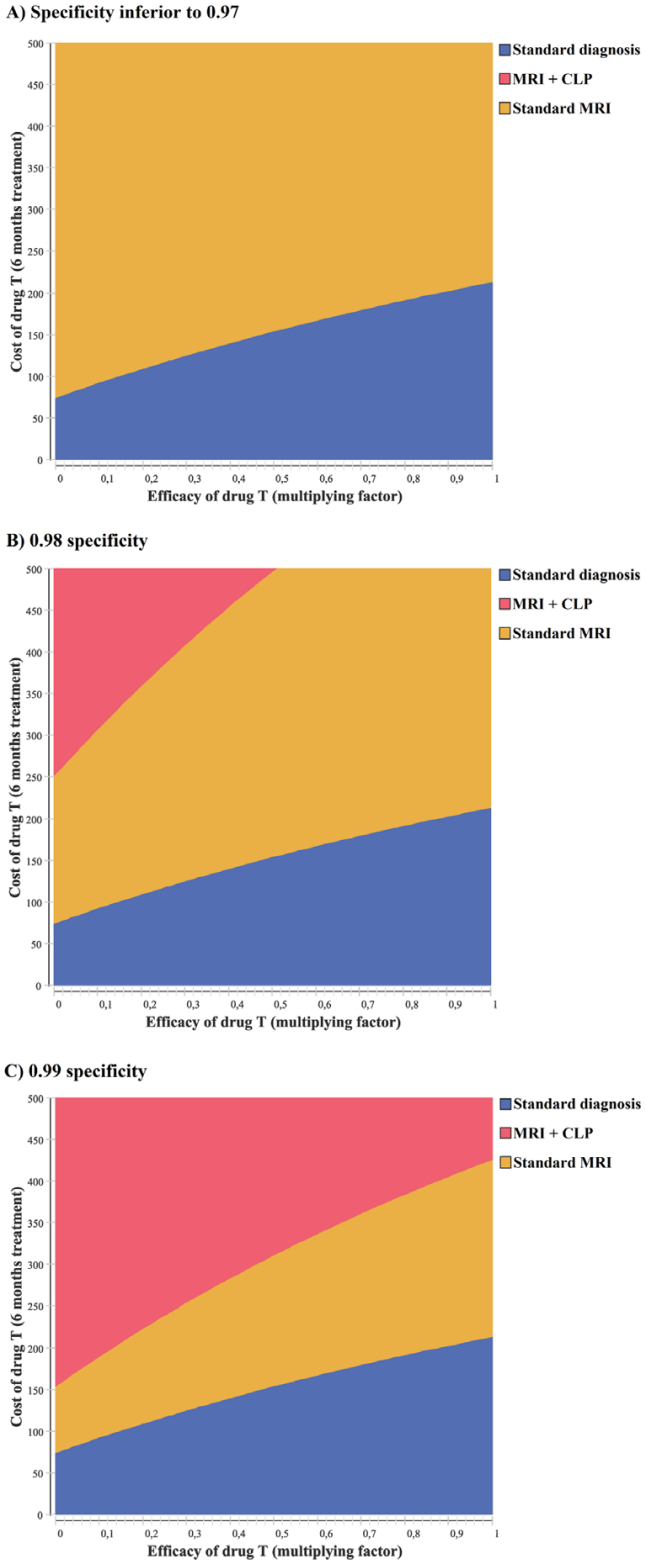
Results of the “screen and treat” (population-wide screening) analysis: multivariate sensitivity analysis. The strategy with maximum net monetary benefit is depicted as a function of the assumed efficacy and cost of the hypothetical new drug T, for assumed specificities of the MRI+CLP diagnosis test between 0.80 and 0.99. The efficacy of treatment T is expressed as a 0-to-1 ratio between assumed probabilities of transition from early stage AD with and without treatment T; 0 corresponds to maximum efficacy and 1 to no efficacy (in the base case, f_T_ = 0.5: 50% reduction). Only costs lower than the base-case cost of 500€ per 6-month treatment are investigated here. (a) specificity for MRI+CLP inferior to 0.97 (including base case). (b) 0.98 specificity for MRI+CLP. (c) 0.99 specificity for MRI+CLP.
